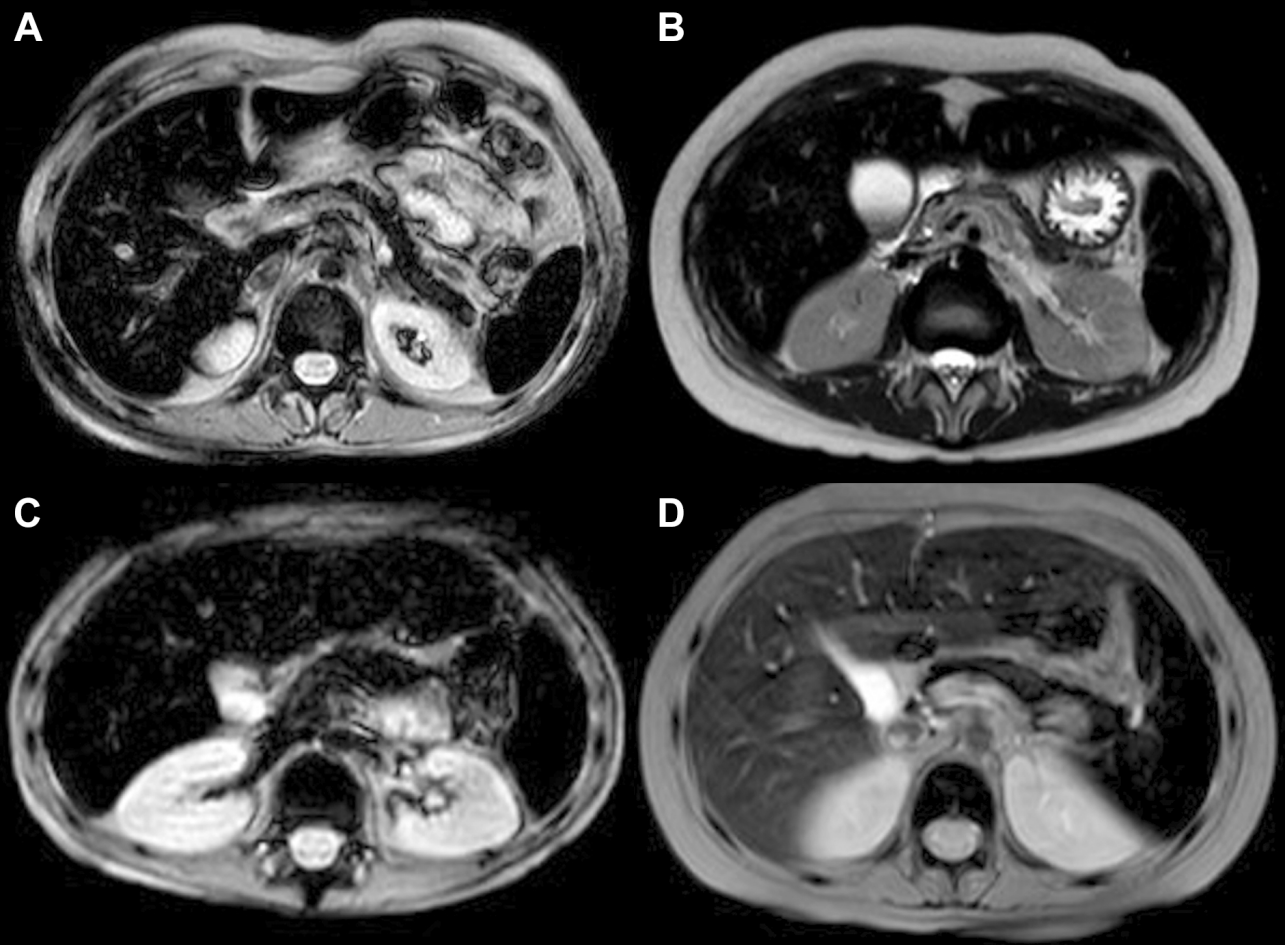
Abdominal MRI T2 FFE (fast field echo) sequences show hypointense liver, spleen, pancreas and bone signal due to abnormal iron deposition (A, B) patients with intense transfusion regimen history; (C) patient with only 5 transfusion before MRI quantification of iron concentration; (D) patient treated with deferasirox: minor liver hyperintensity compared to spleen, pancreas and bone intensity.
